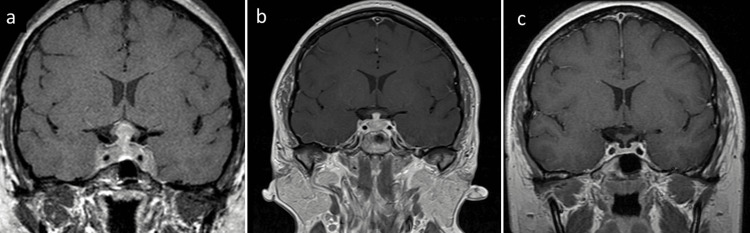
MRI findings at diagnosis and follow-up Magnetic resonance imaging of the pituitary gland (coronal section, T1 weighted, post-gadolinium). (a) Symmetric enlargement of the pituitary gland with bilateral extension to the cavernous sinuses (predominantly on the left, and thickening of the pituitary stalk (10.9 mm) at diagnosis (b) significant reduction in the size of the pituitary gland and pituitary stalk (4.05 mm) with less extension to the cavernous sinuses during follow-up, one month after treatment; (c) normal size of the pituitary gland and pituitary stalk (1.44 mm) fifteen months after treatment.
